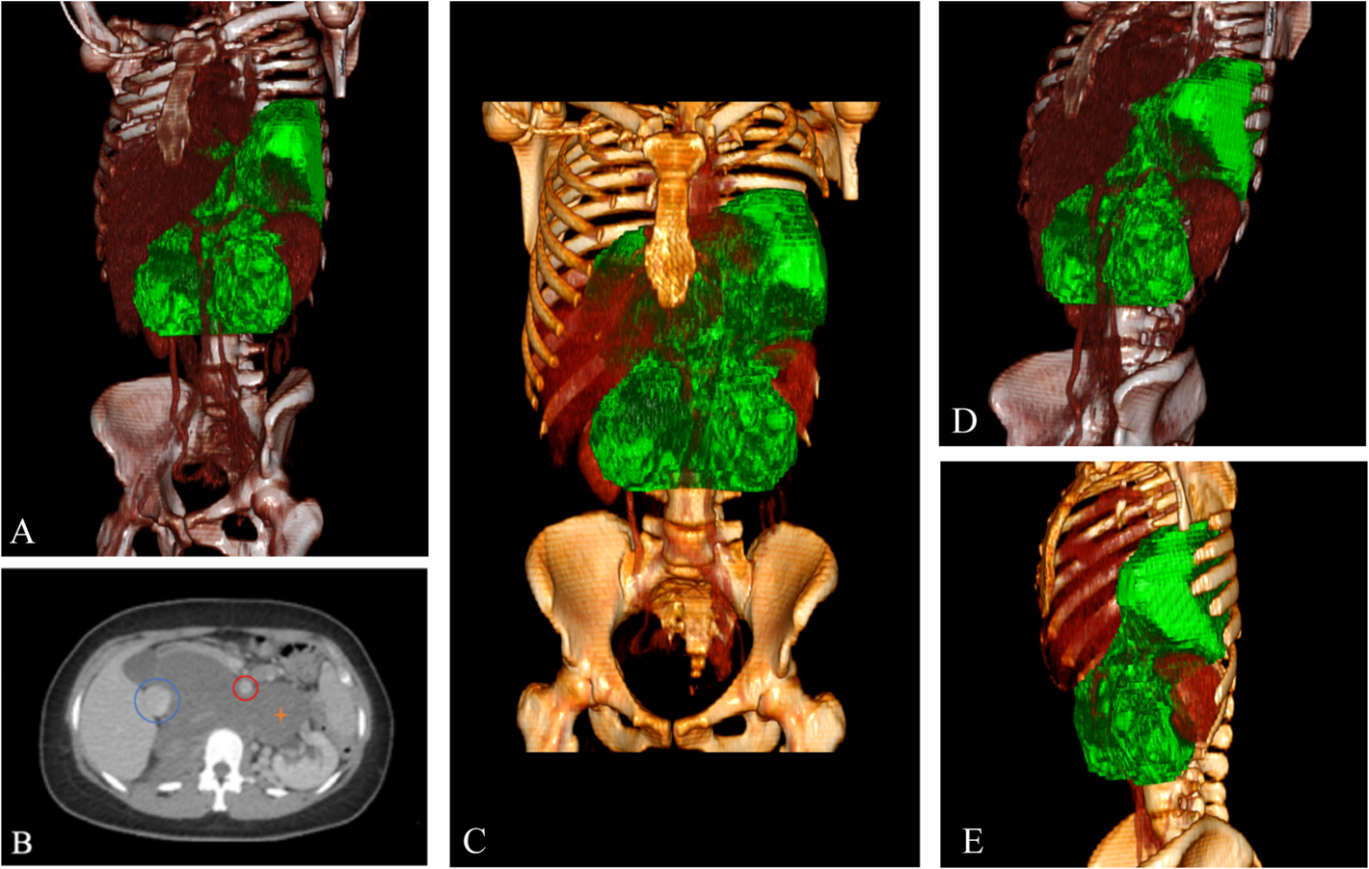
CT images and 3D reconstruction. The GN (green) extends from the retroperitoneal space through the aortic hiatus into the posterior mediastinum and surrounds (a, c–e) and displaces (b) Inferior vena cava , aorta , tumor
